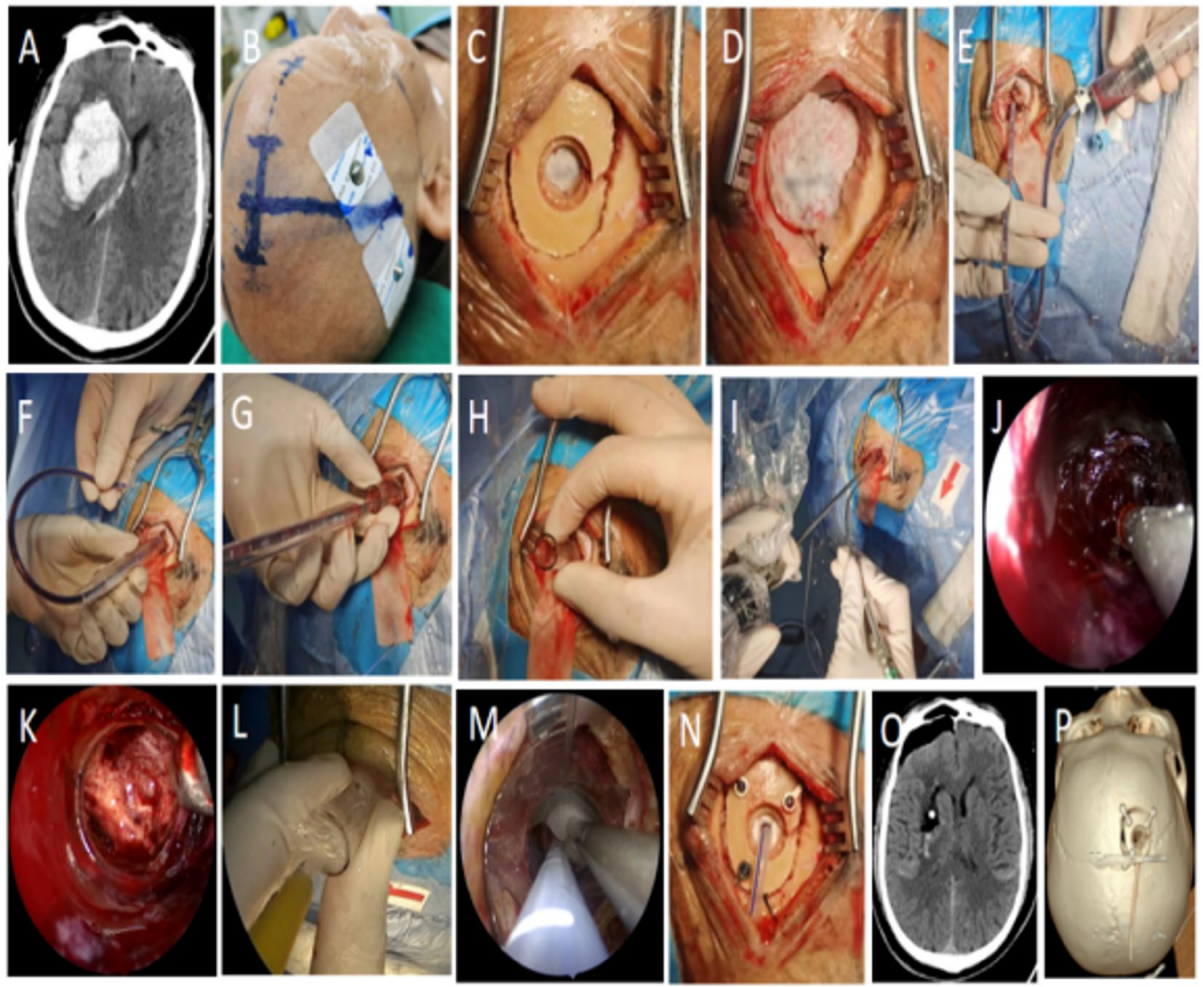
Surgical procedure for right basal ganglia hemorrhage. (A) Head CT showing right basal ganglia hemorrhage. (B) Surgical path planning based on head CT and skin mark. (Skin marker location was decided based on preoperative CT reconstruction, marking the entry point and trajectory on the scalp to guide the insertion angle and depth.) (C-D) Surgical incision 5 cm and bone window 2.5 cm x 3.0 cm. (E) Guide-wire drainage tube aspiration of dark blood confirming position within hematoma cavity; 10–20 ml aspirated for gradual ICP reduction. (F-G) Sequential insertion of primary and secondary dilator sleeves over the drainage tube; subsequent removal of drainage tube and primary sleeve. (H-I) Secondary/Working sleeve in place; neuroendoscope and suction introduced. (J-K) Hematoma evacuation; exposed white matter after evacuation. (L-M) Irrigation via working sleeve; no active bleeding observed; hemostatic material placed under endoscopic vision; drainage tube placement. (N) Bone flap replacement and fixation. (O-P) Postoperative head CT showing satisfactory evacuation of right basal ganglia hematoma; 3D reconstruction showing right frontal keyhole approach.
